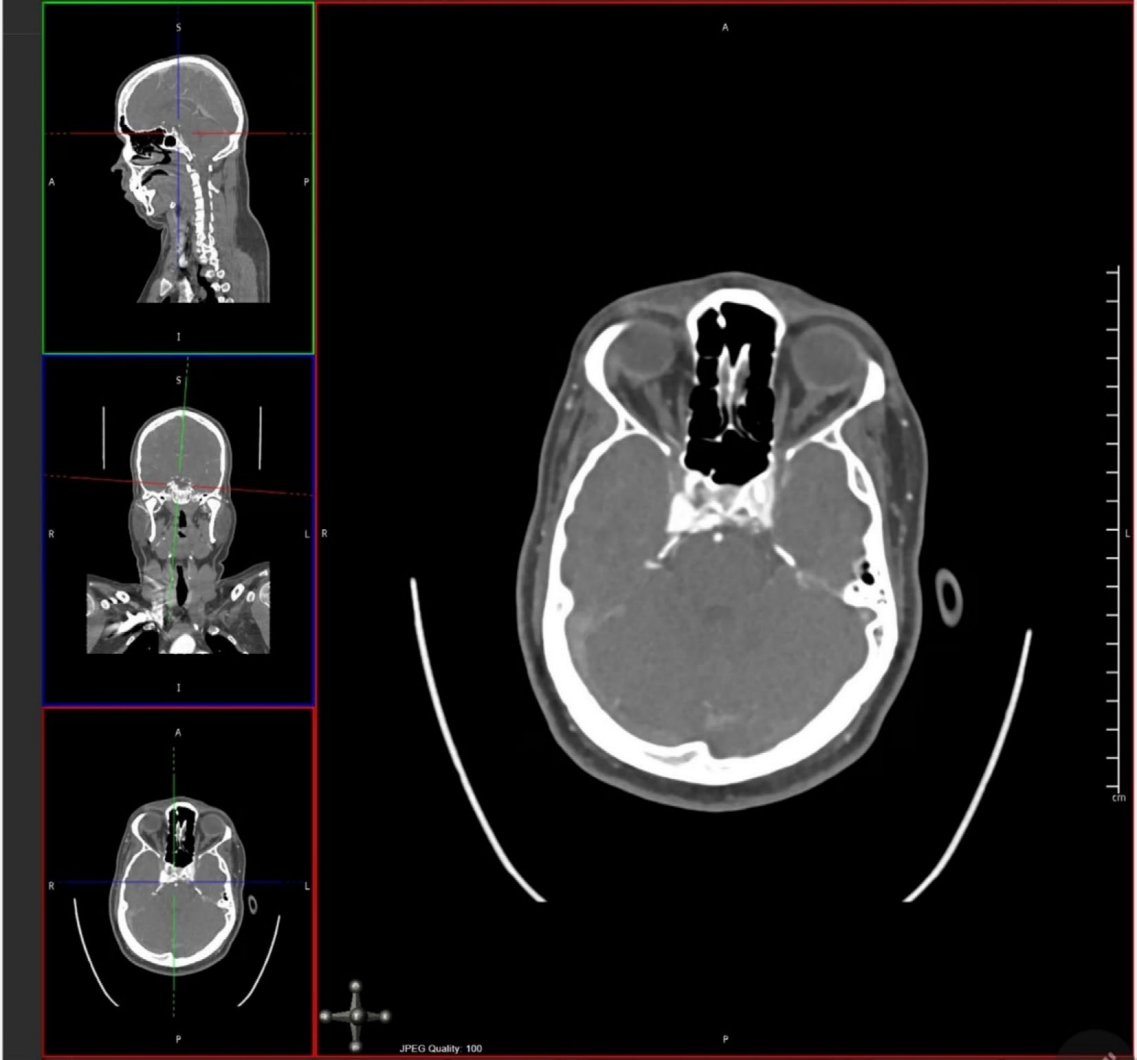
Showing multiplanar reconstructed CT images of the brain with angiographic contrast, axial, coronal, and sagittal reconstructions demonstrate markedly dilated superior ophthalmic veins and engorged cavernous sinuses bilaterally, consistent with high‐flow arteriovenous shunting.
